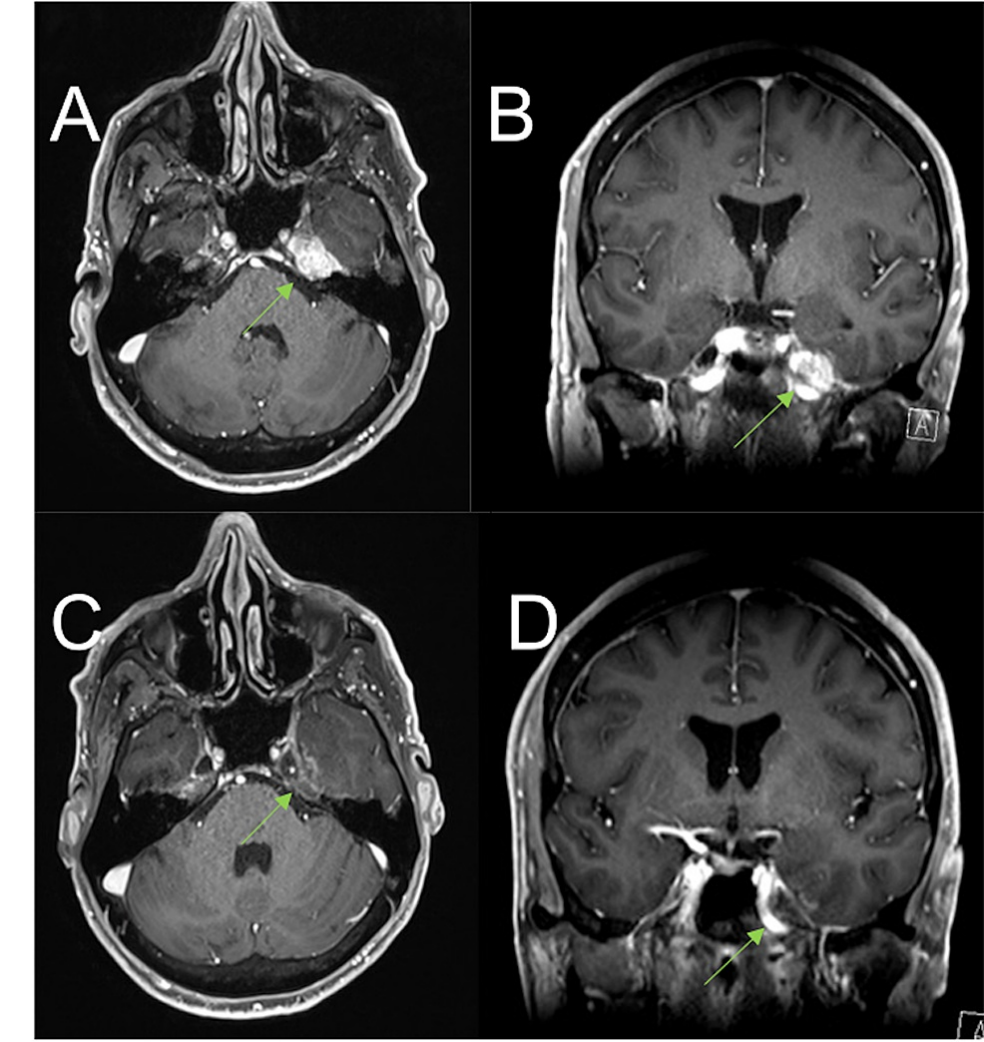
(A, B) MRI brain (T1-weighted gadolinium-enhanced) taken on October 2019. Follow-up study 29 months post-GK SRS and one month prior to repeat GK SRS with (A) transverse and (B) coronal views displaying progressive disease in left Meckel's cave extending to the cavernous sinus. (C, D) MRI brain (T1-weighted gadolinium-enhanced) taken on August 2020. Follow-up study nine months after repeat GK SRS with (C) transverse and (D) coronal views displaying mild early post-treatment effect enhancement in the adjacent temporal lobe. MRI, magnetic resonance imaging; GK, Gamma Knife; SRS, stereotactic radiosurgery.
